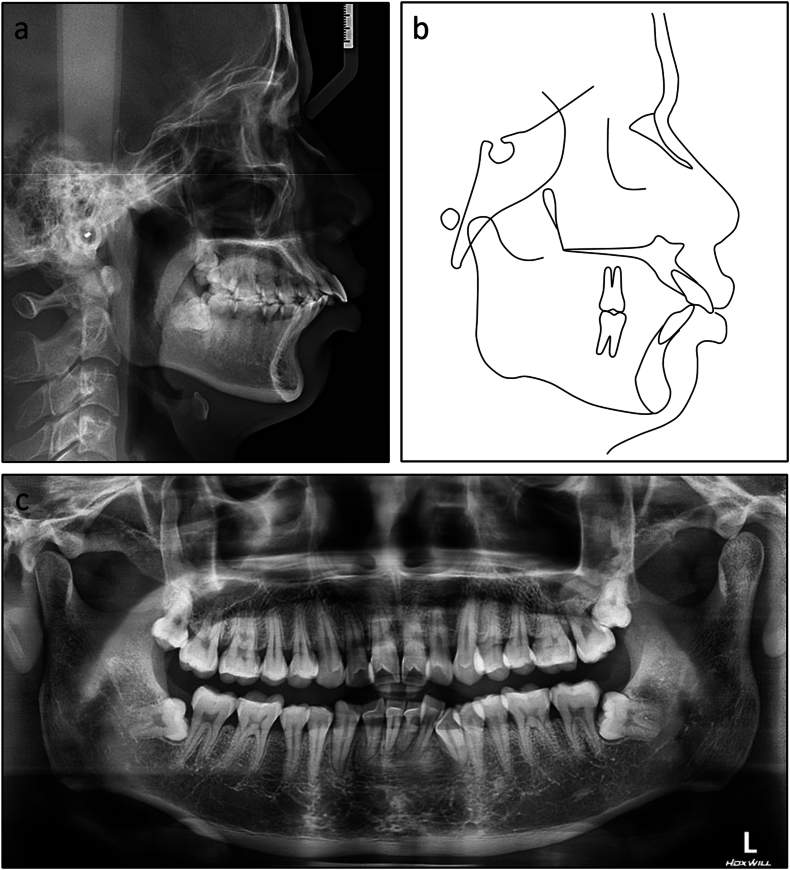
Pretreatment radiographs and cephalometric tracing of Case 2. (a) Cephalometric radiograph. (b) Cephalometric tracing. (c) Panoramic radiograph.
